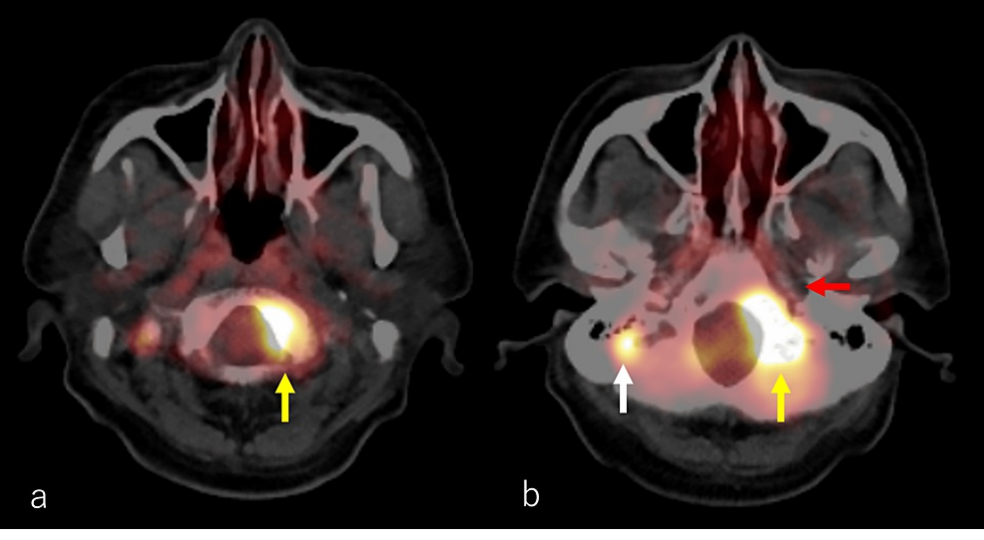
Fluorodeoxyglucose-positron emission tomography. The image showed fluorodeoxyglucose (FDG) uptake extending from the left side of the oblique plateau to the left side of the occipital bone (a, b; yellow arrows). There appeared to be no obvious extension toward the jugular foramen (b; red arrow). A continuous uptake pattern was also observed in the right occipital bone (b; white arrow).
